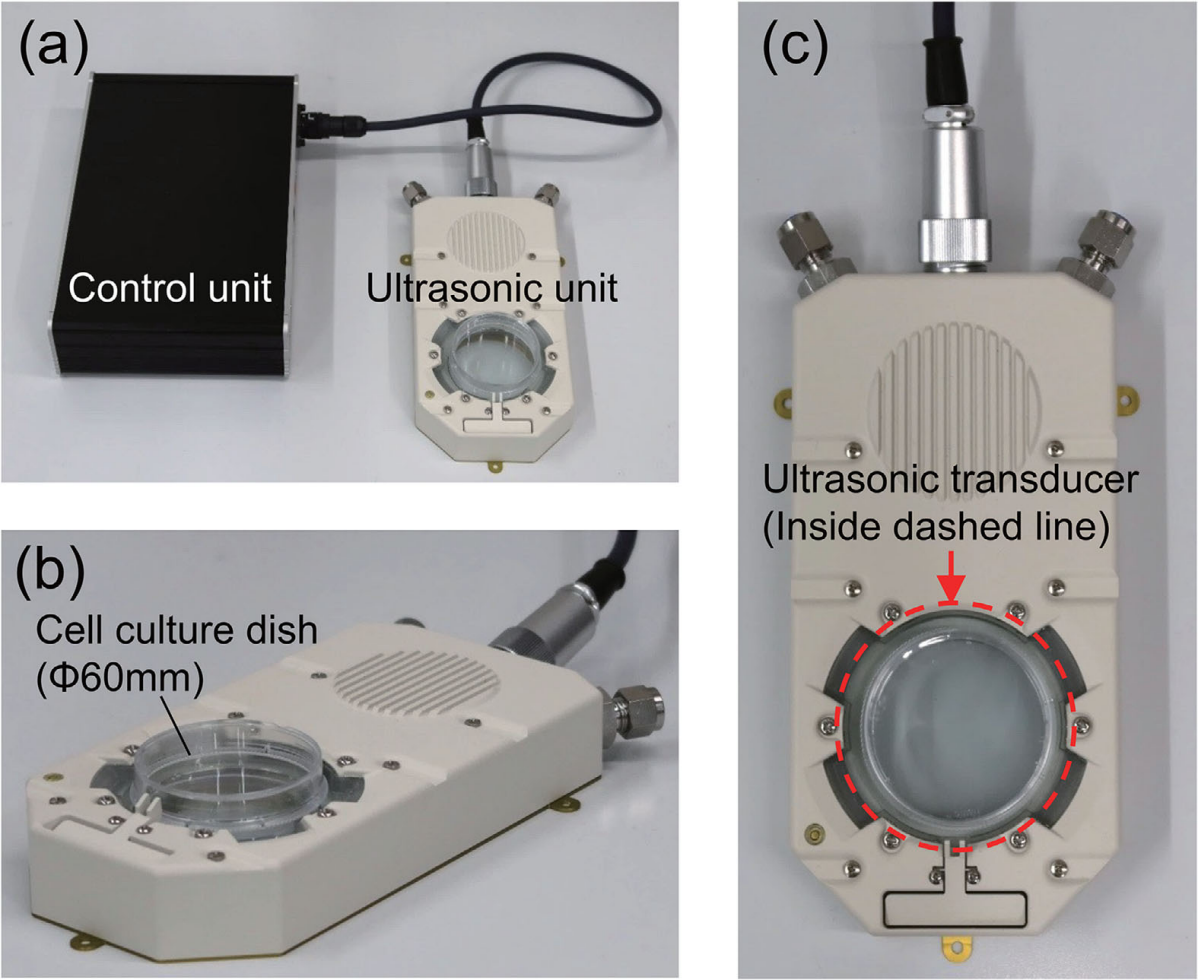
Ultrasound detachment device. a) Overall view of the detachment device from above with control unit and ultrasonic unit. b) Bird view of the ultrasonic unit with 60‐mm dish. c) Upper view of ultrasonic unit showing the ultrasonic transducer at the bottom of the 60‐mm dish.
